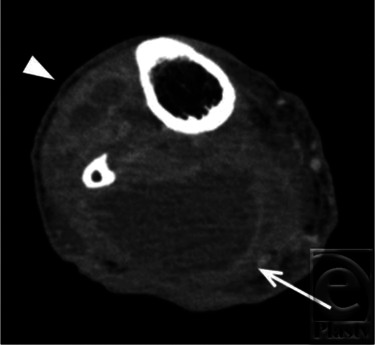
Contrast computed tomographic scan shows fluid collection in both the posterior segment (white arrow) and the anterior segment (white arrowhead).
